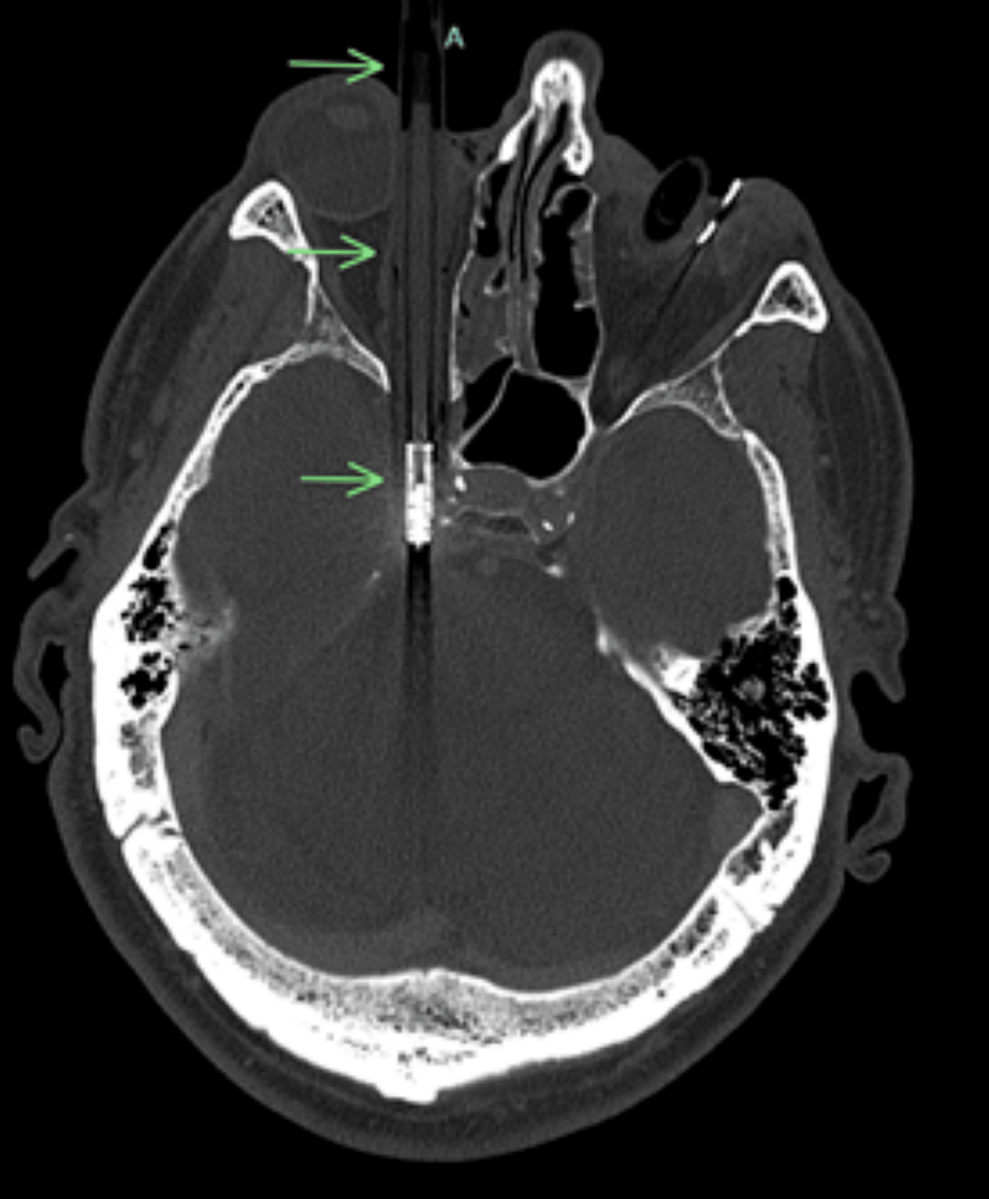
Axial computed tomography image demonstrating a ballpoint pen penetrating the right orbit, extending posteriorly toward the cavernous sinus region.
